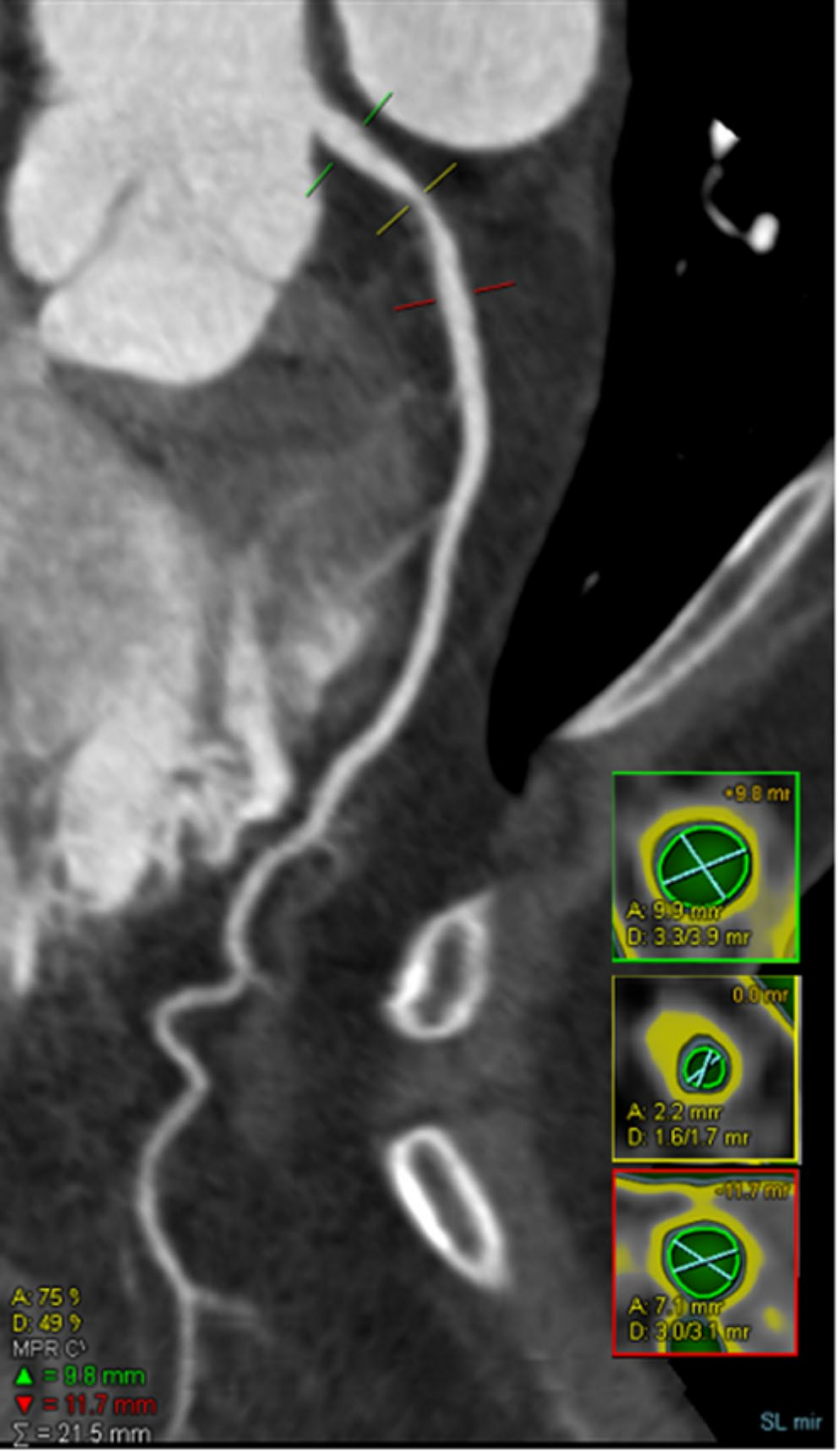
Fifty-eight-year-old male with chest pain for 4 weeks. Non-calcified plaque component and severe stenosis are observed in proximal circumflex artery by CTA. Cross-sectional images show high-risk plaque and stenosis features
